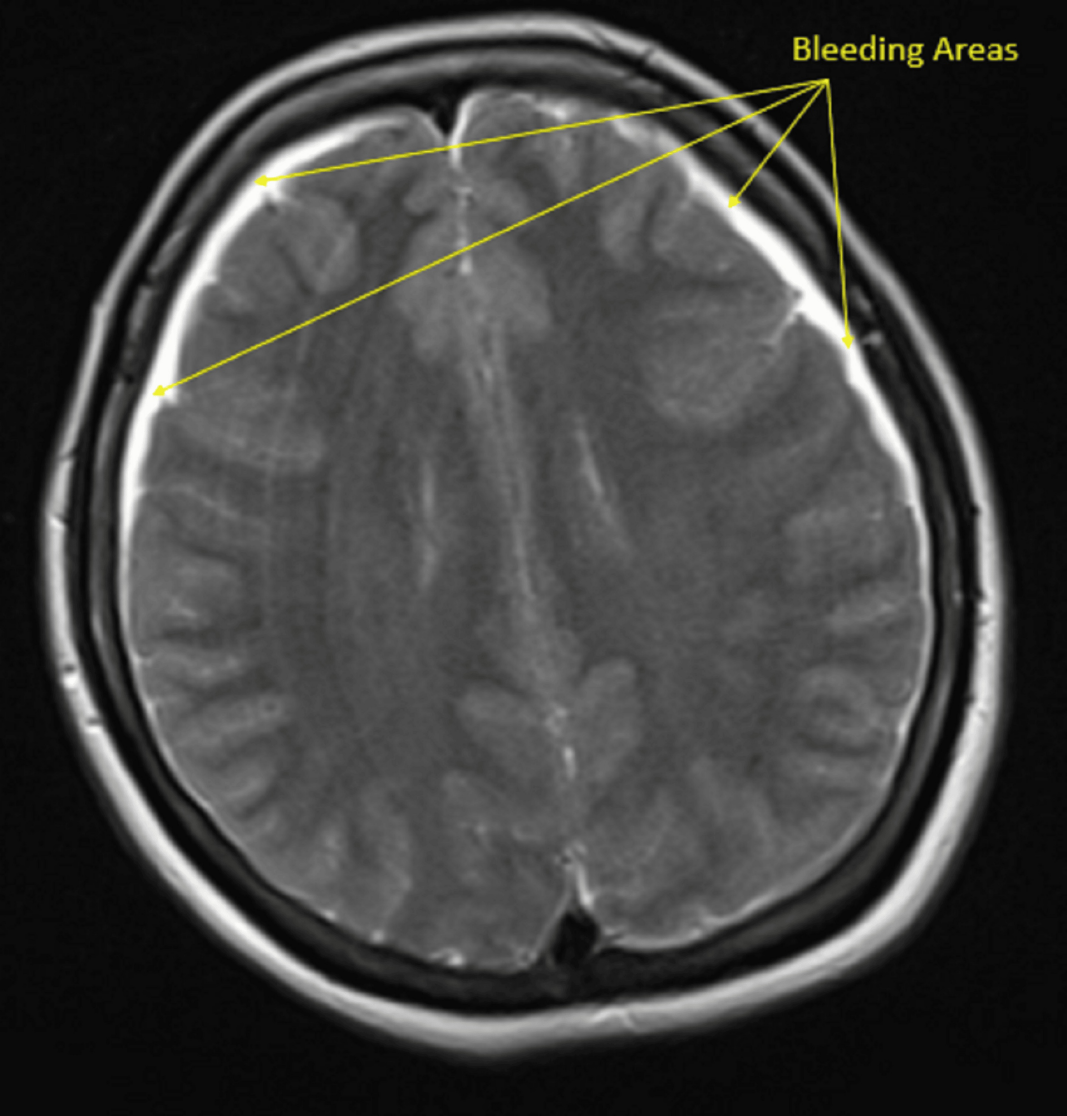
Bilateral subdural hematoma observed in the magnetic resonance image (MRI) obtained on the fifth postoperative day The arrows indicate the bleeding areas.
